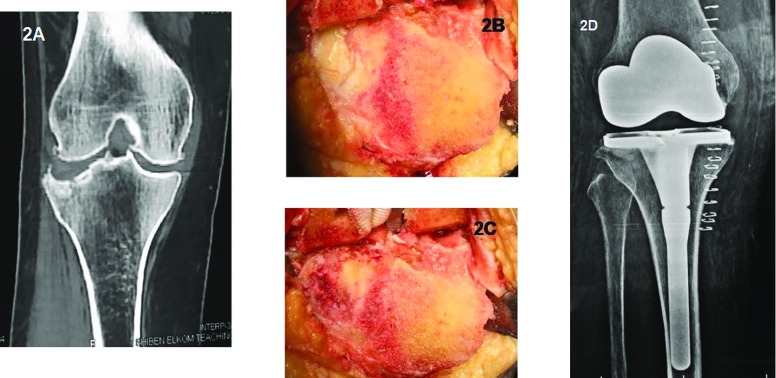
(A) Preoperative CT scan showing lateral depression of the tibial plateau and medial joint arthritic changes. (B) Intra-articular photo after proximal tibial cut. Lateral depressed articular surface with remaining cartilage covering. (C) Peripheral bone defect after removal of the depressed articular cartilage. (D) Postoperative X-ray with a stem added to the tibial tray and cement filling of the lateral defect.
